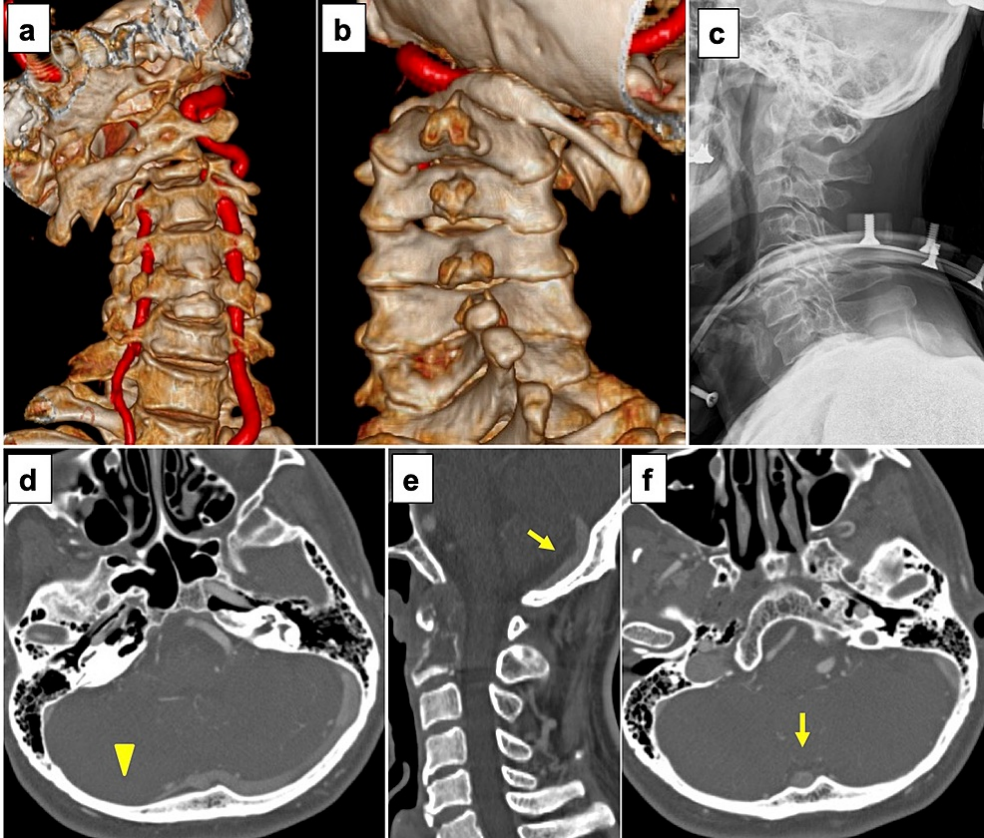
Preoperative images (a, b) Three-dimensional reconstruction of computed tomography (CT) image showing a pathological odontoid fracture and atlantoaxial dislocation. (c) Lateral radiograph showing reposition after closed reduction with a halo-vest. (d) Contrast-enhanced axial CT showing an absent right transverse sinus (arrowhead). (e), (f) Contrast-enhanced CT showing a prominent occipital venous sinus (arrows).
